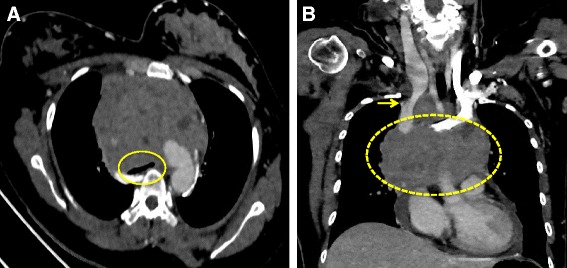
a CT scan showing the mediastinal mass severely compressing the trachea (*solid line circle*). b Enhanced CT scan showing a large anterior-superior mediastinal mass [113 × 87 × 68 mm (length, height, width; *dotted line circle*)] compressing the superior vena cava (*yellow arrow*)
